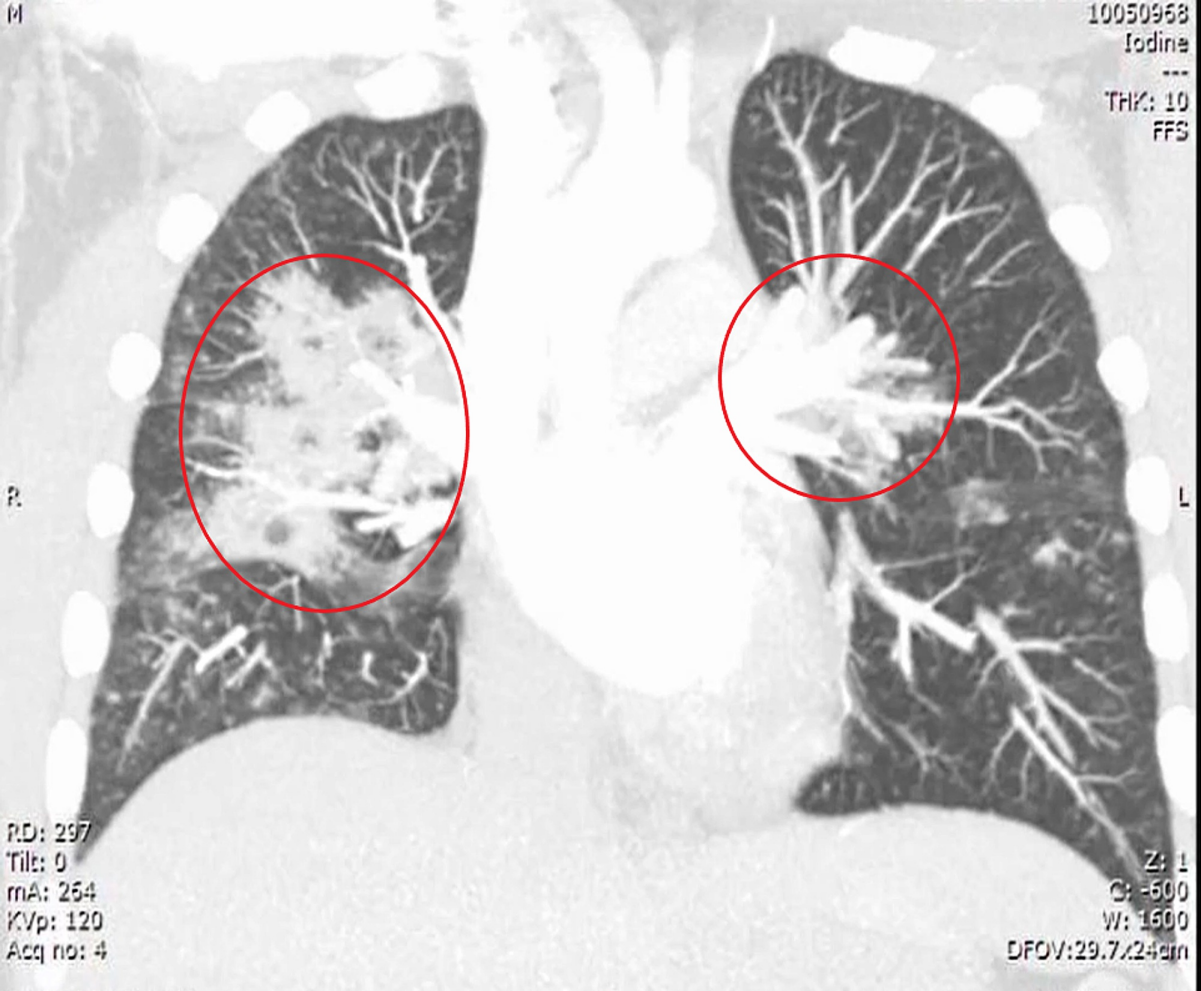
CT chest single slice coronal view maximum intensity projection image demonstrates bilateral perihilar focal consolidation, left (circle) greater than right (circle).
